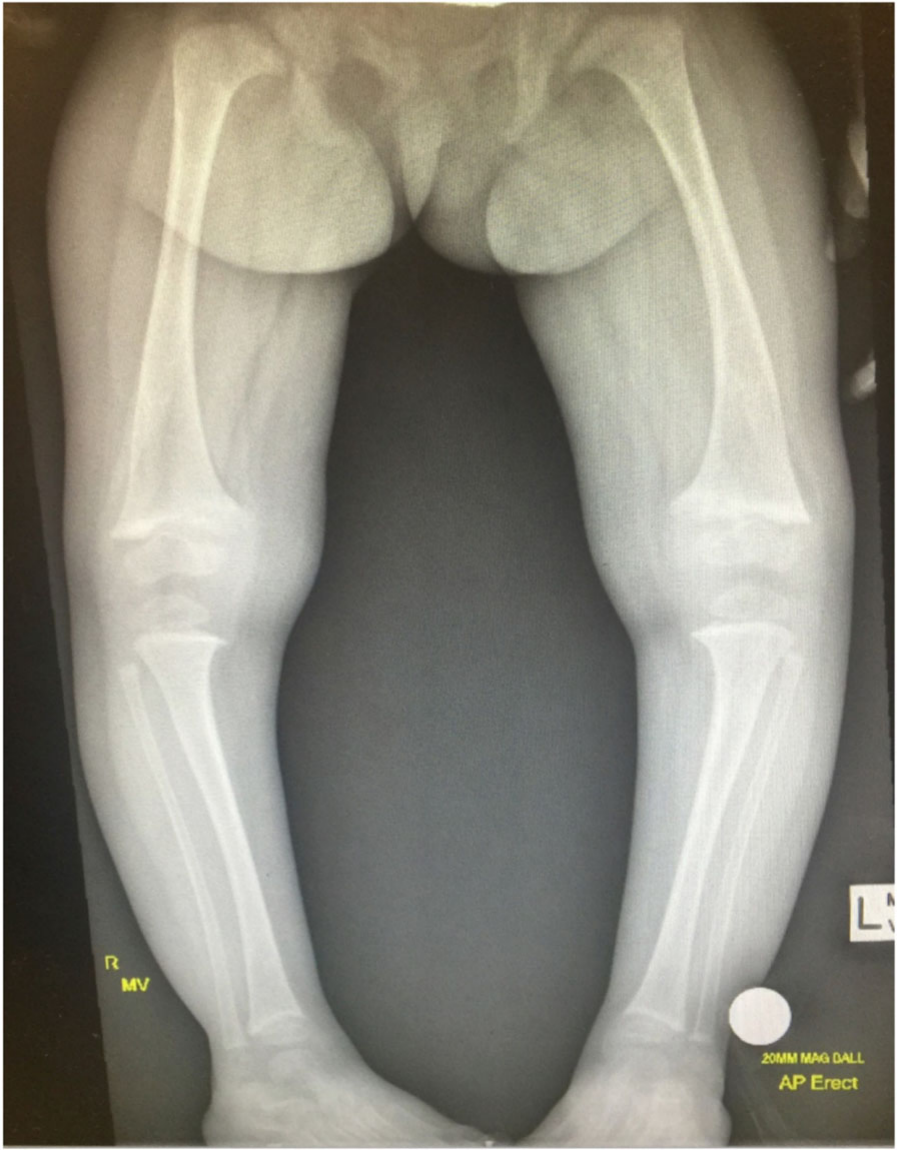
X-ray of the lower extremities of a child with nutritional rickets. Classic radiological signs of rickets are seen including cupping, fraying, and widening of the growth plates and bowing of the diaphyses
